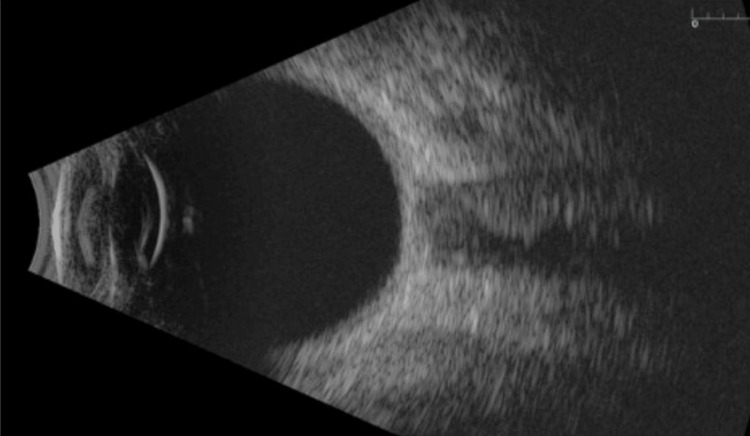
Ultrasound B-scan demonstrating normal globe structure in the left eye
